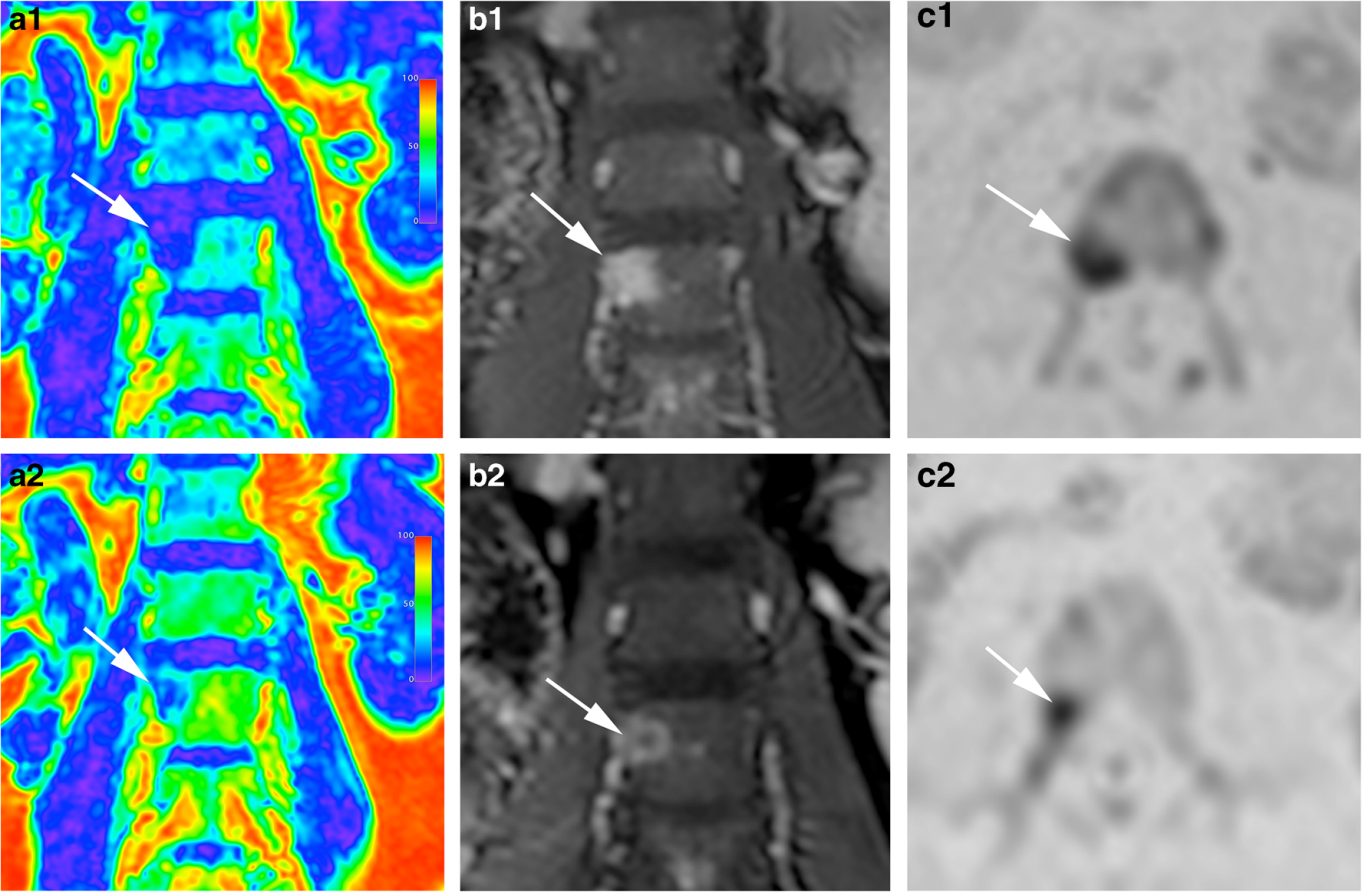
Representative images of the whole-body MRI scan of a 52-year-old female participant prior to (*a1*–*c1*) and following two cycles (*a2*–*c2*) of induction PAD (bortezomib, doxorubicin, dexamethasone) chemotherapy. *a1*, *a2* coronal signal fat fraction map; *b1*, *b2* coronal post-contrast water-only mDixon; *c1*, *c2* Axial *b*
